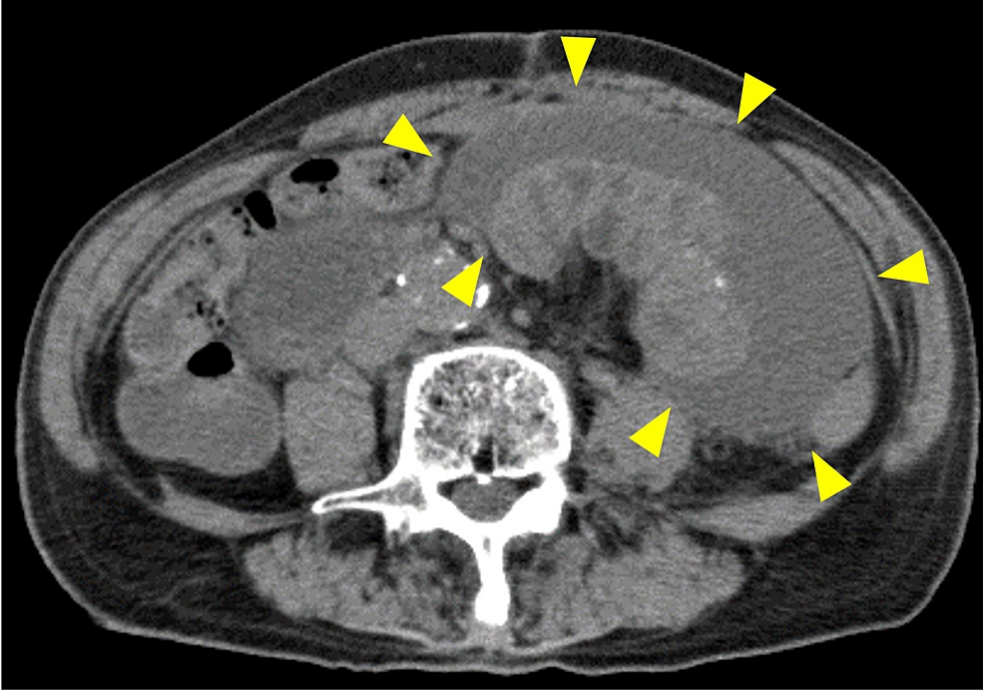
Abdominal computed tomography Abdominal CT showed a mass of jejunum in the encapsulated area with ascites and calcified nodules.
